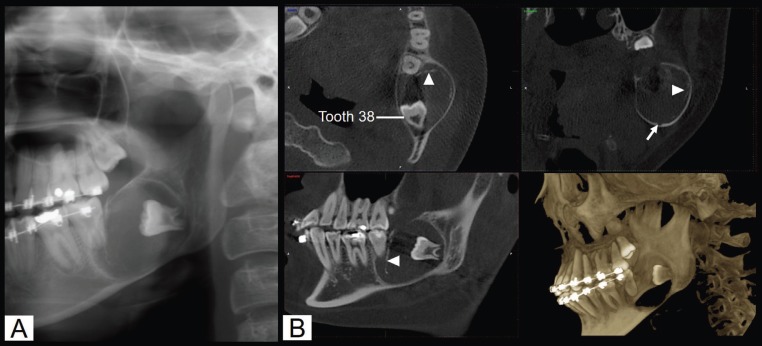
A) A cropped panoramic image shows a well-defined unilocular lesion with a corticated border, extending from the mesial root of the second molar to the ramus. A thin radiopaque band is noted at the anterior portion. B) Cone beam computed tomography (CBCT) images including axial, coronal, sagittal, and volume rendering reformation reveal a unilocular hypodensity lesion with buccal and lingual cortical bone expansions. A thinning of the inferior border of the mandible is observed. The inferior alveolar nerve canal (arrow) is displaced inferiorly. The radiopaque foci (arrowheads) are seen at the anterior portion.
